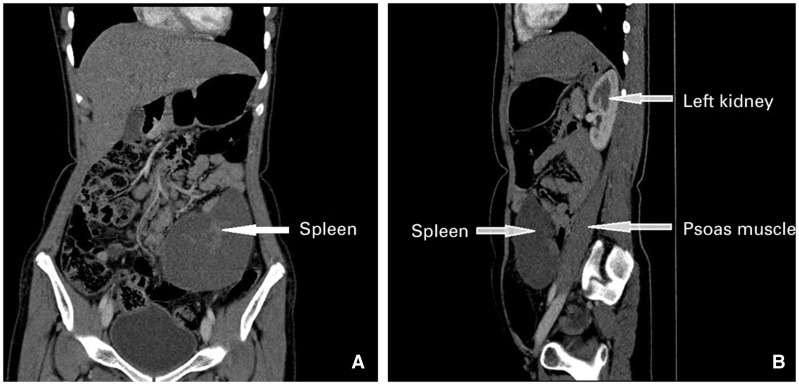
Contrast-enhanced CT images of abdomen. (A) Coronal reformatted image shows that the spleen has migrated from the left hypochondrium and is ectopically located in the left lumbar and iliac region (marked with an arrow). (B) Sagittal reformatted image shows that the spleen is located inferior to the left kidney.
